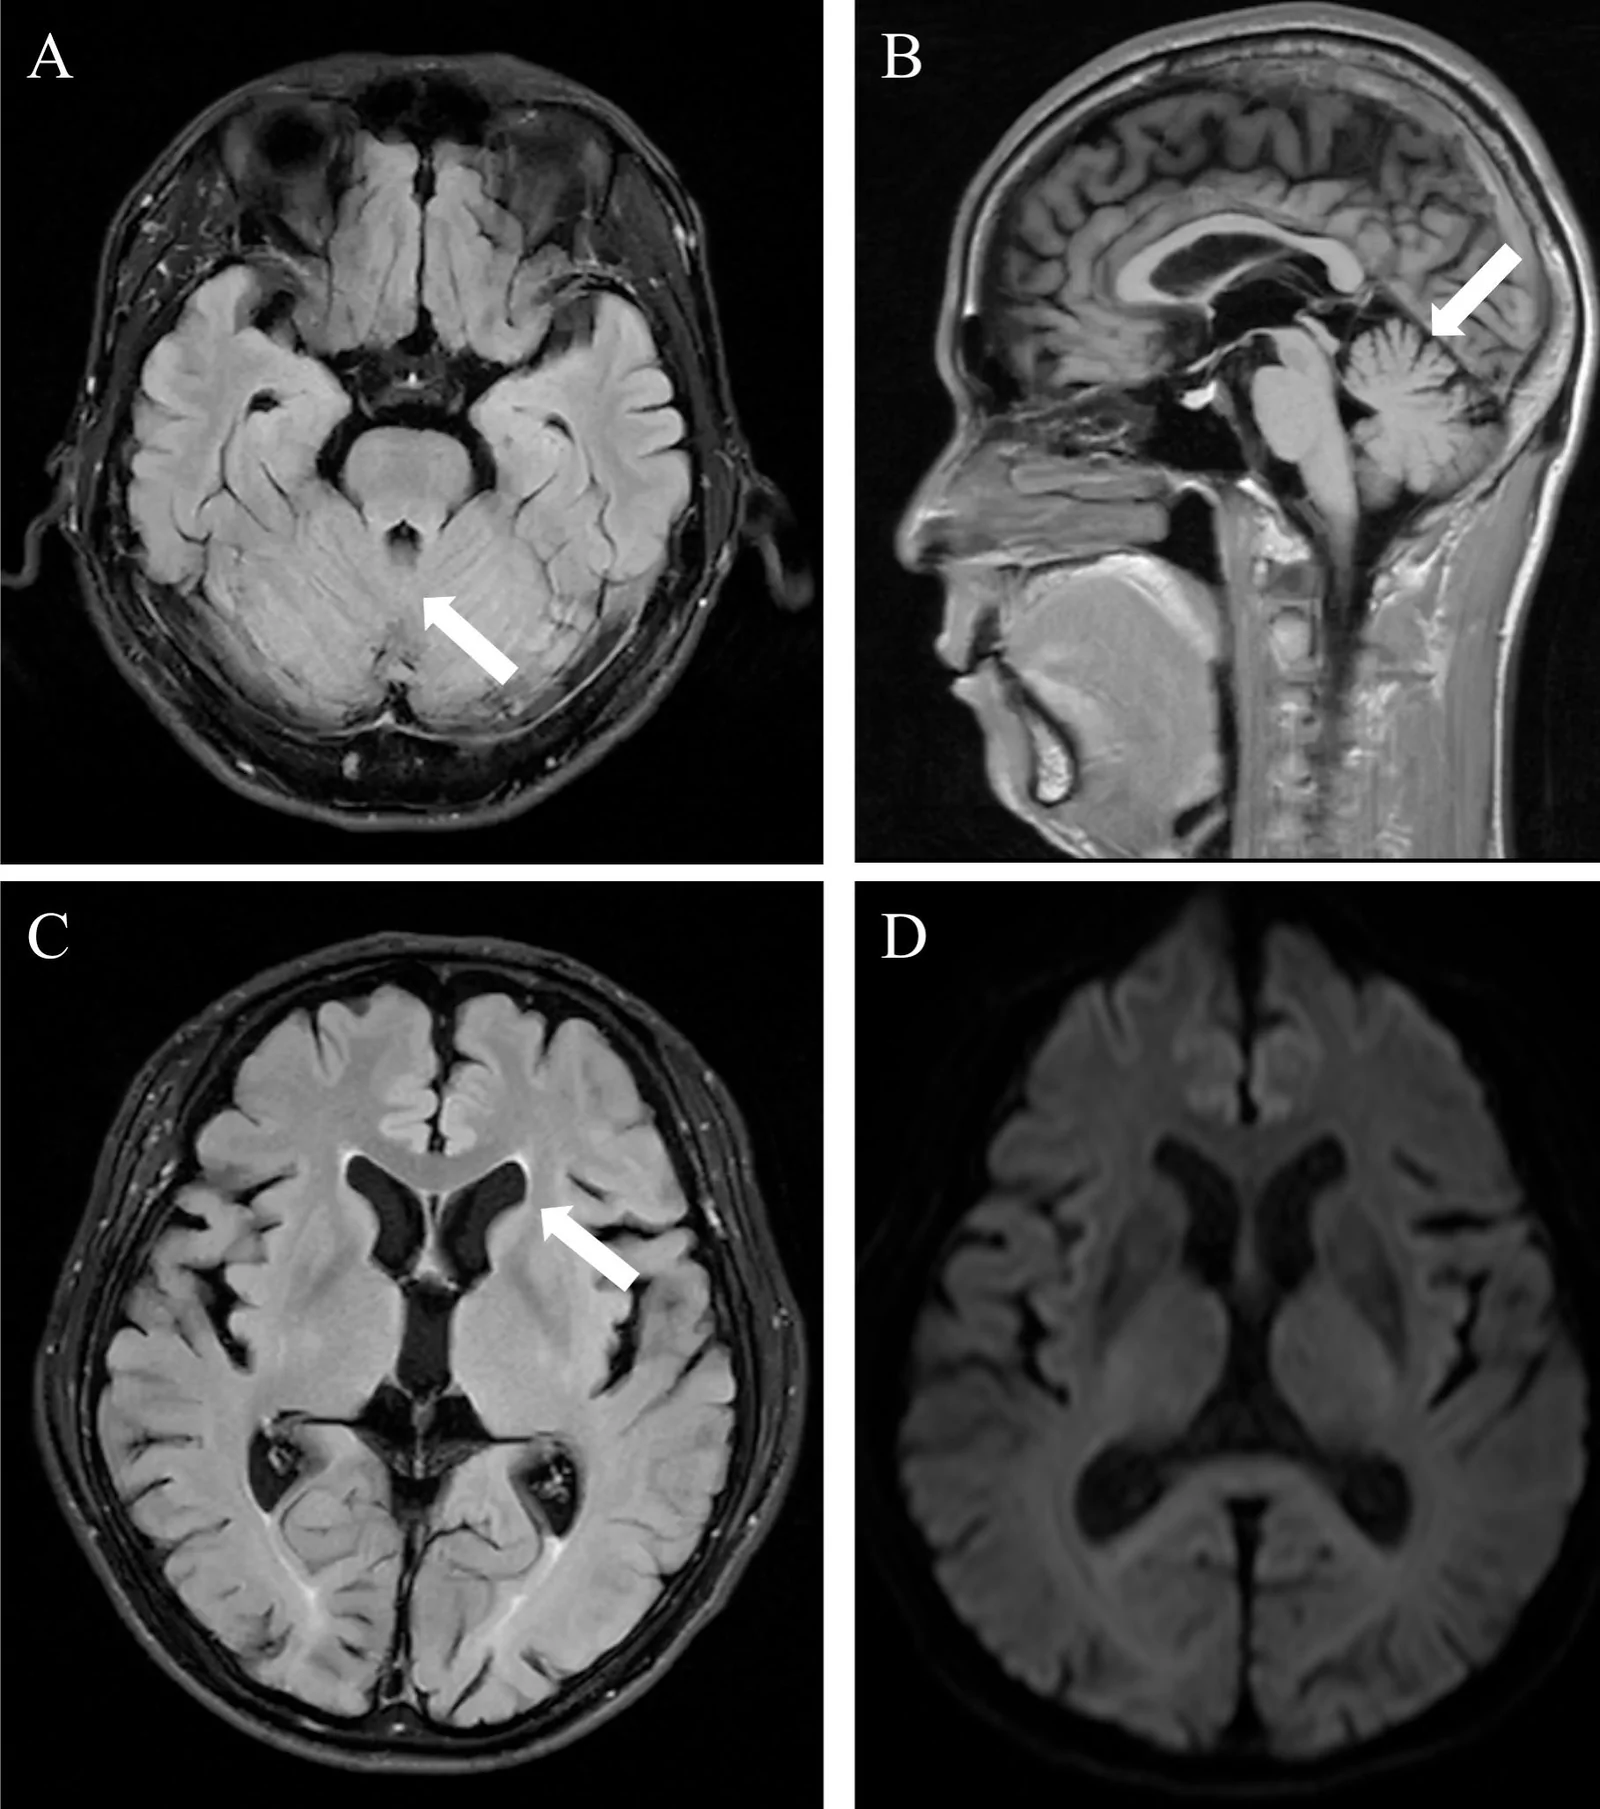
Serial brain MRI findings. Panels A–C: Scans performed at a referring tertiary hospital three months prior to admission. Panel A: Axial FLAIR image showing mild atrophy of the cerebellar vermis (arrow). Panel B: Sagittal T1-weighted image demonstrating mild cerebellar cortical atrophy (arrow). Panel C: Axial FLAIR image revealing enlargement of the frontal horns of the lateral ventricles (arrow). Panel D: Axial Diffusion-Weighted Imaging (DWI) acquired on the day of admission, showing no abnormal high signal intensity.
